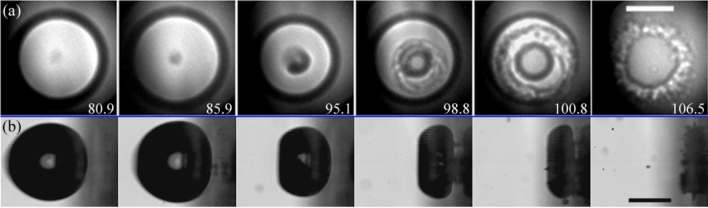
a X-ray holographic images in parallel view of the jet impacting the rigid boundary at $$\gamma =0.84\pm 0.03$$. Here the bubble is located around $$r_b=2.8$$ mm from the centre of the plate (i.e. $$\alpha _r=2.4$$). The scale bars represent $$250\,{\upmu \text {m}}$$, the numbers indicate time in $$\upmu {s}$$. b Optical images in side view corresponding to the frames in (a), taken perpendicular to the glass disc and orthogonal to the x-ray beam direction
